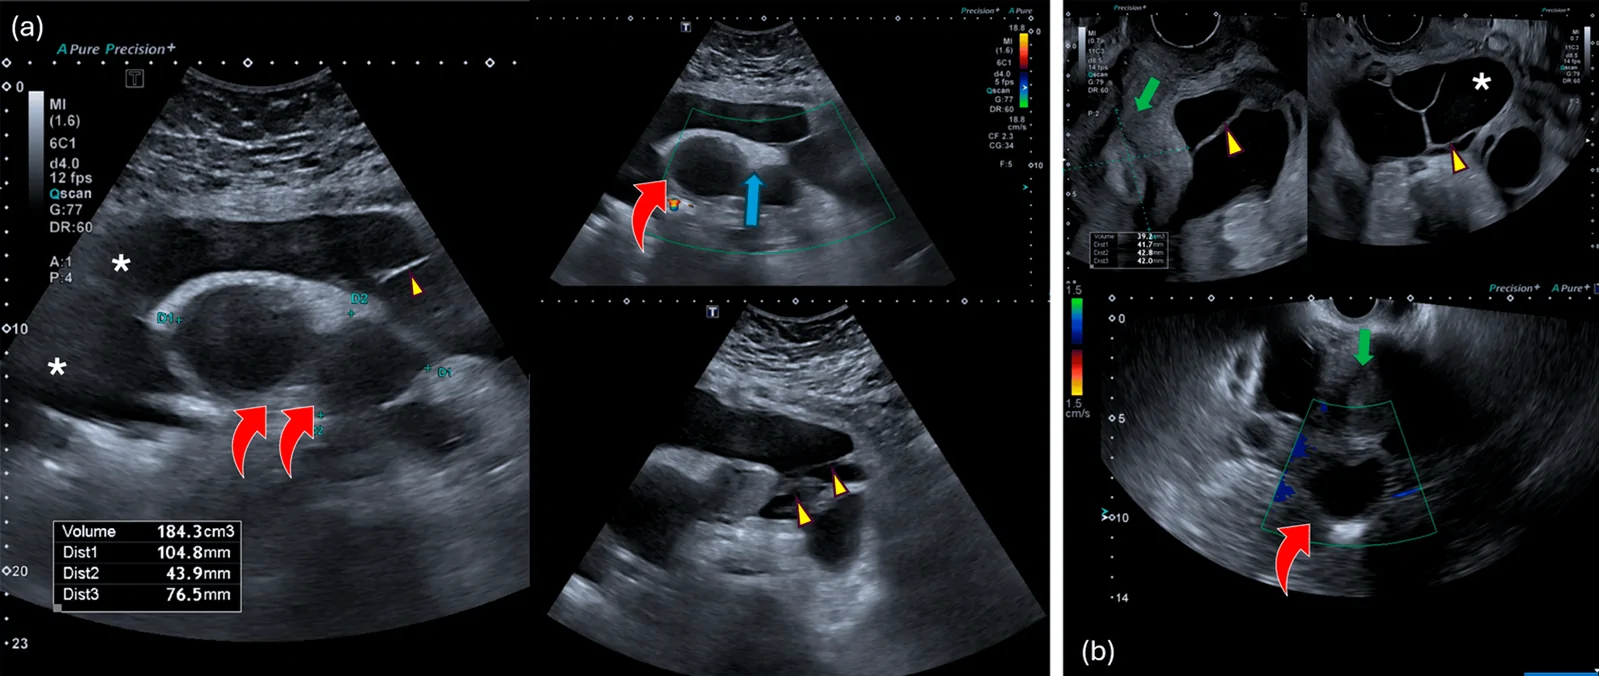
(a) Transabdominal gynecological ultrasound and (b) transvaginal ultrasound Lobulated collection vs. cystic adnexal lesion (red arrows). No flow on color Doppler (blue arrow). Abundant ascites with a non-free appearance, multiple septa (yellow arrowhead) in pelvic recesses, and partially particulate content (asterisks). The uterus appears normal (green arrow).
